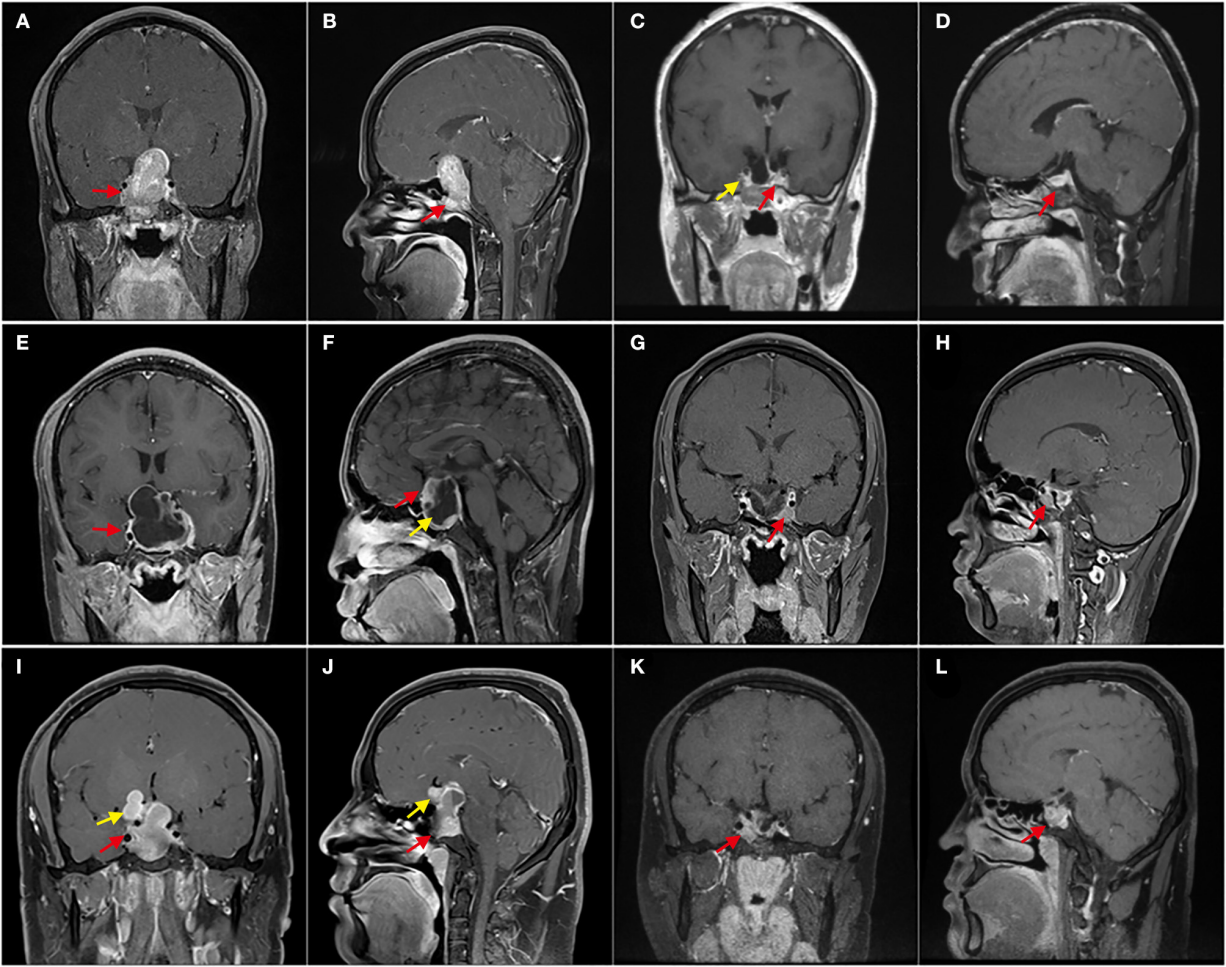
Enhanced T1 MRI with different resection degrees. (A–D) Patient 1, Preoperative MRI showed that the tumor invaded the sphenoid sinus downward and severely squeezed the right cavernous sinus (red arrow); 3 months after the operation, MRI showed that there was no residue in the right cavernous sinus (yellow arrow), and the normal pituitary was located on the left side of the sellar area (red arrow); It is gross-total resection. (E–H) Patient 2, E shows GPA with giant cystic, and F shows solid components (red arrow) and cystic components (yellow arrow); (G,H), 3 months after the operation, MRI shows a few residual tumors in the left cavernous sinus (red arrow). With a residual of about 5%, which was subtotal resection. (I–L) Patient 3, the tumor was lobulated, extended to the anterior and suprasellar (yellow arrow), and invaded the sphenoid sinus (red arrow); (K,L) shows that 3 months after the operation, the residual tumor was mainly located in the left cavernous sinus (red arrow), and the residual was about 25%, which was partially resectioned.
